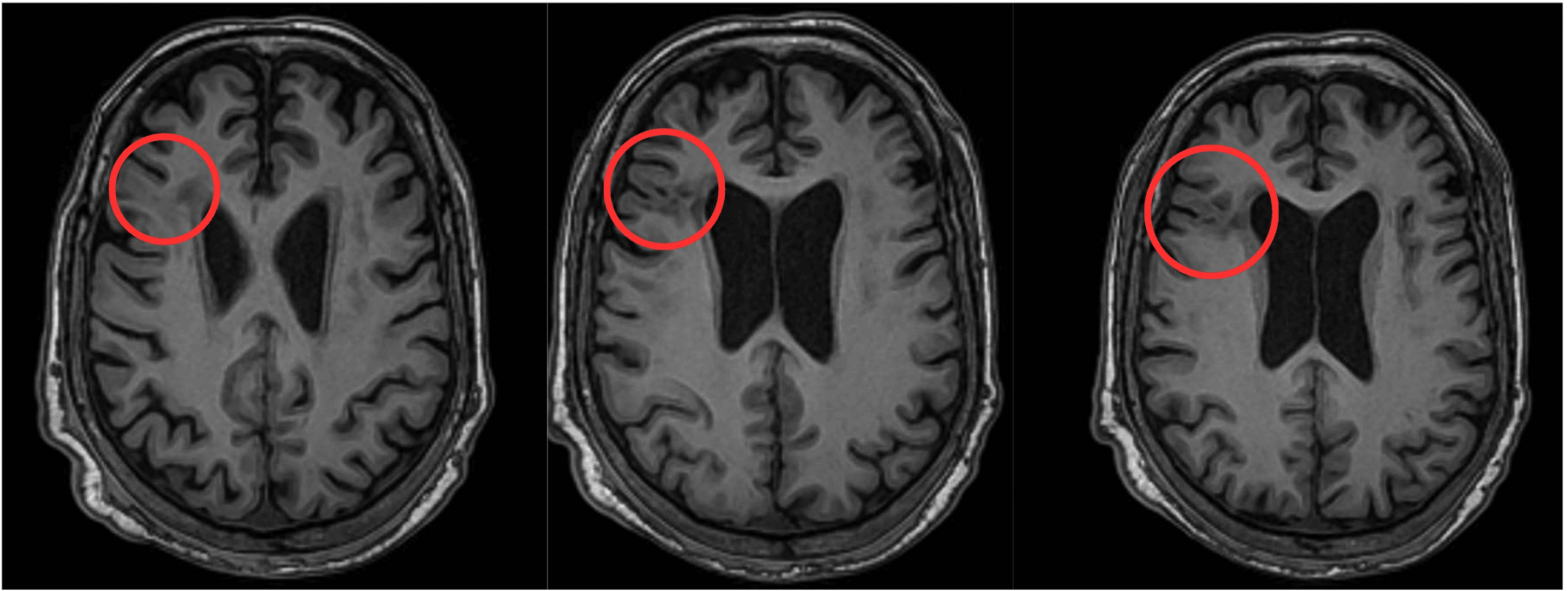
(Left to right) Brain MRI with HARNESS protocol Axial 3D BRAVO reconstruction with T1-weighted image consistent with focal cortical dysplasia (likely type 2b) in the right inferior frontal gyrus. HARNESS: Harmonized Neuroimaging of Epilepsy Structural Sequences
